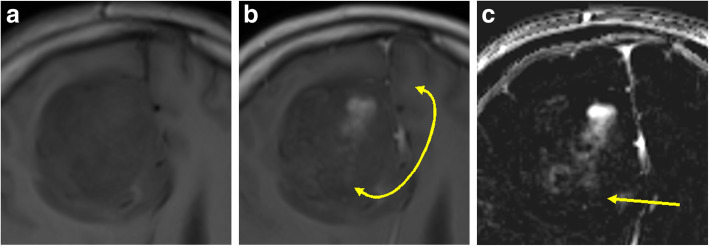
The quantitative enhancement (QE) analysis system analyses both unenhanced (a) and contrast-enhanced (b) T1-weighted images allowing accurate quantification of enhancement in regions that are much darker than normal appearing white matter (arrow on the QE map in c)
